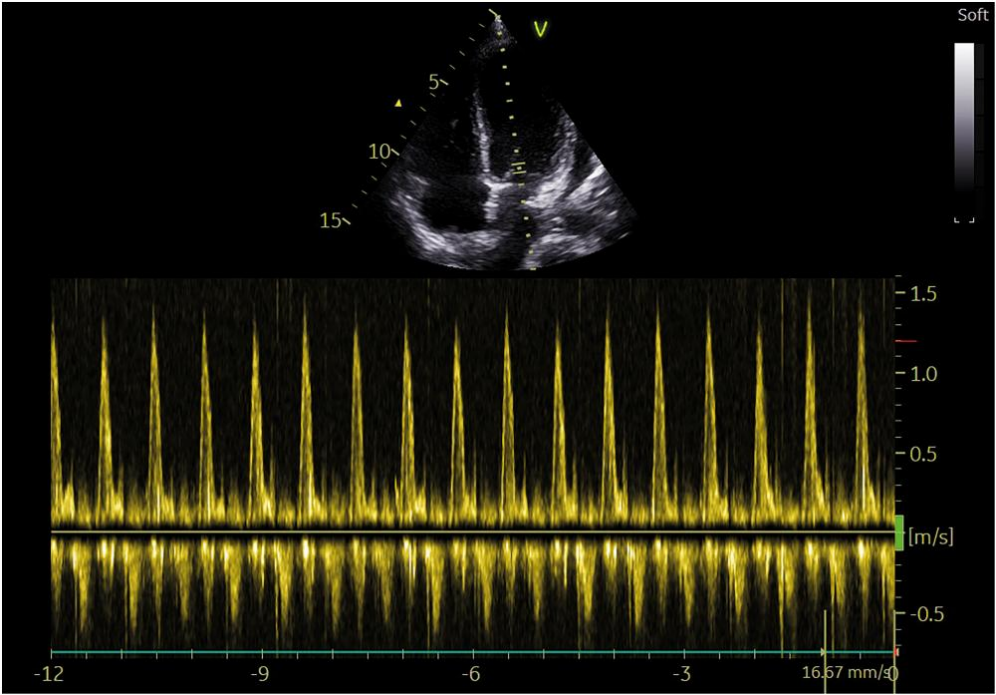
Transmitral pulse-wave Doppler. Transmitral pulse-wave Doppler showing no respiratory variability.
